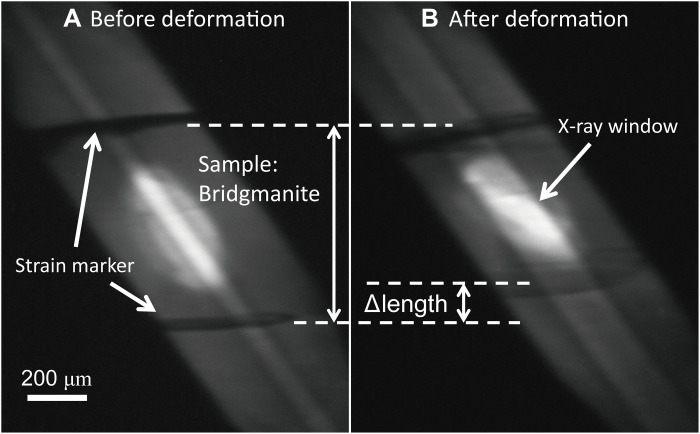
X-ray radiographs of bridgmanite deformation experiments in run KM19 in the D111 apparatus. (A) Before deformation. (B) After deformation. Total strain reached 21.0%. Delta length is the shortened length of the sample by uniaxial deformation.
